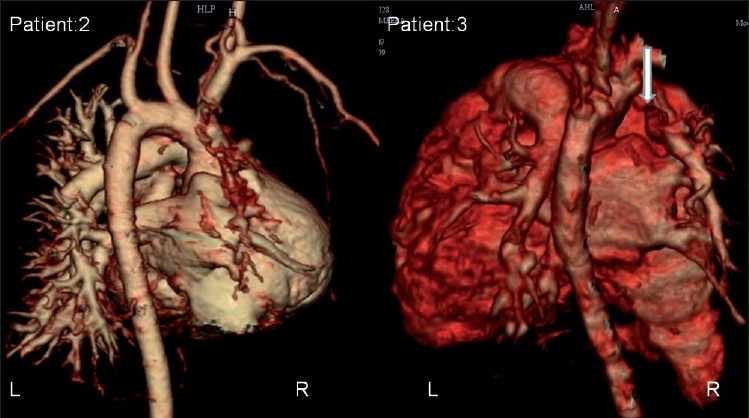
Multidetector computed tomography images of patient# 2 and patient# 3. The heart is seen from its posterior aspect. The right pulmonary artery is absent and the hilar pulmonary artery is diminutive (arrow). R, right side; L, left side
